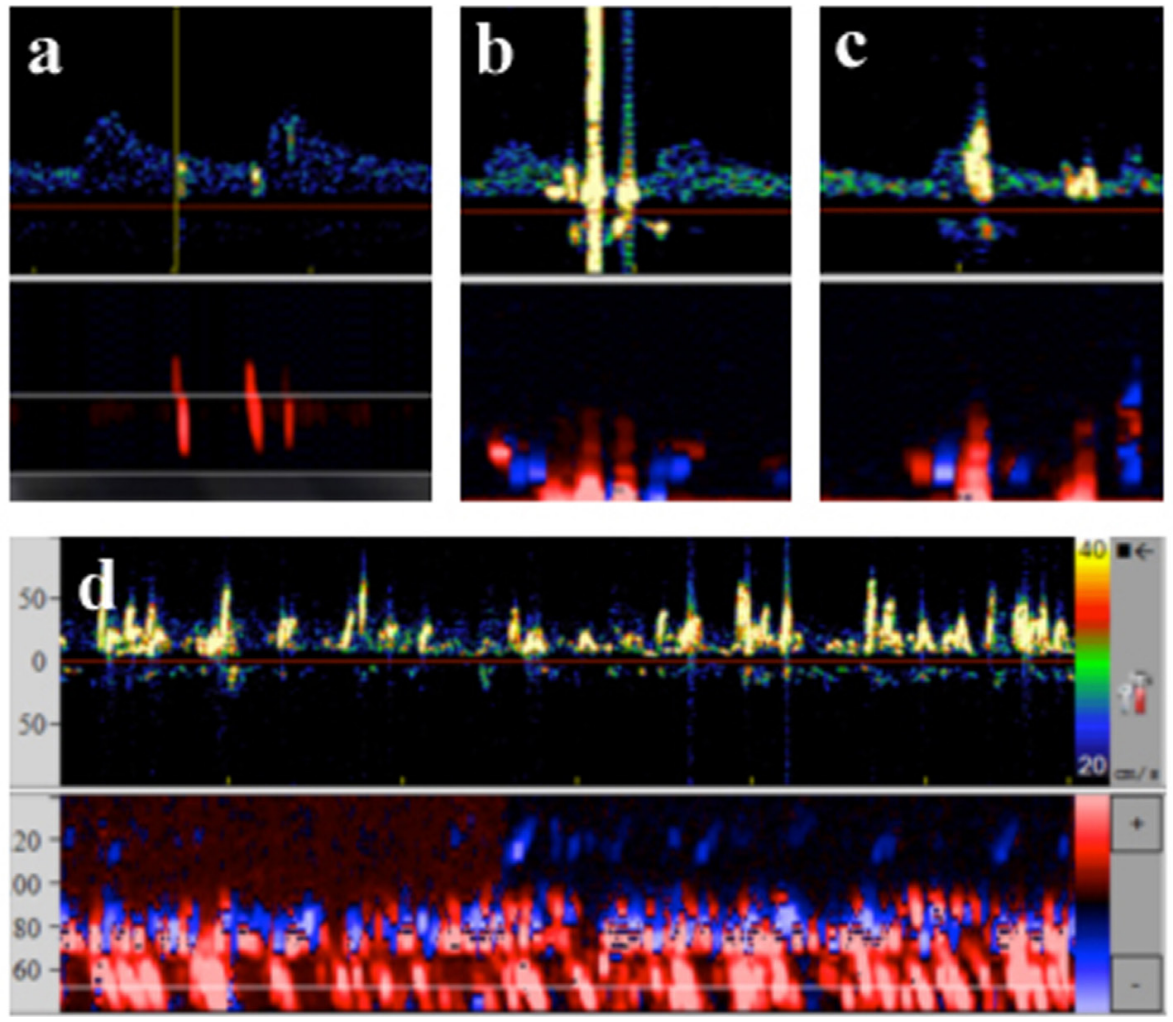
Transcranial Doppler data and typical characteristics of microembolic signal. (A) Solid emboli: stays within the Doppler spectrum, multigating confirms movement through different depths. (B) Gaseous emboli: exceeds the Doppler spectrum, biphasic, multigating reveals signals at one depth only. (C) Equivocal emboli: exceeds the Doppler spectrum, but unidirectional, multigating reveals signals at one depth only. (D) Thrombembolic shower: signals move at low velocity, continuous embolic activity, oblique multigated signals reveal movement at different depths.
